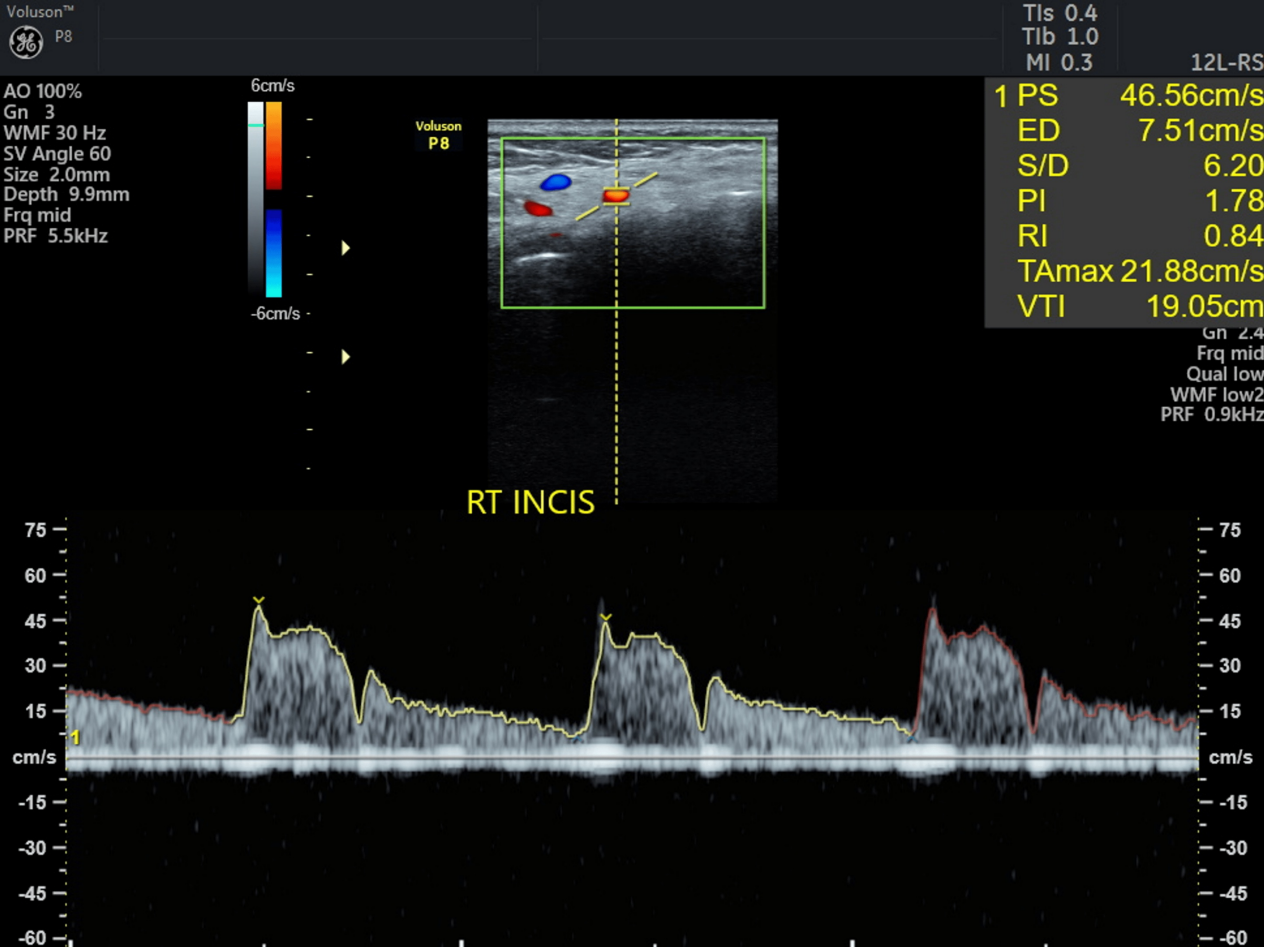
Ultrasound Doppler flowmetry of CAF + Mucograft on the fifth day. CAF: coronally advanced flap, CTG: connective tissue graft, PS: peak systolic velocity, ED: end-diastolic velocity, PI: pulsatility index, RI: resistive index, VTI: velocity time integral, RT INCI: right incisor.
